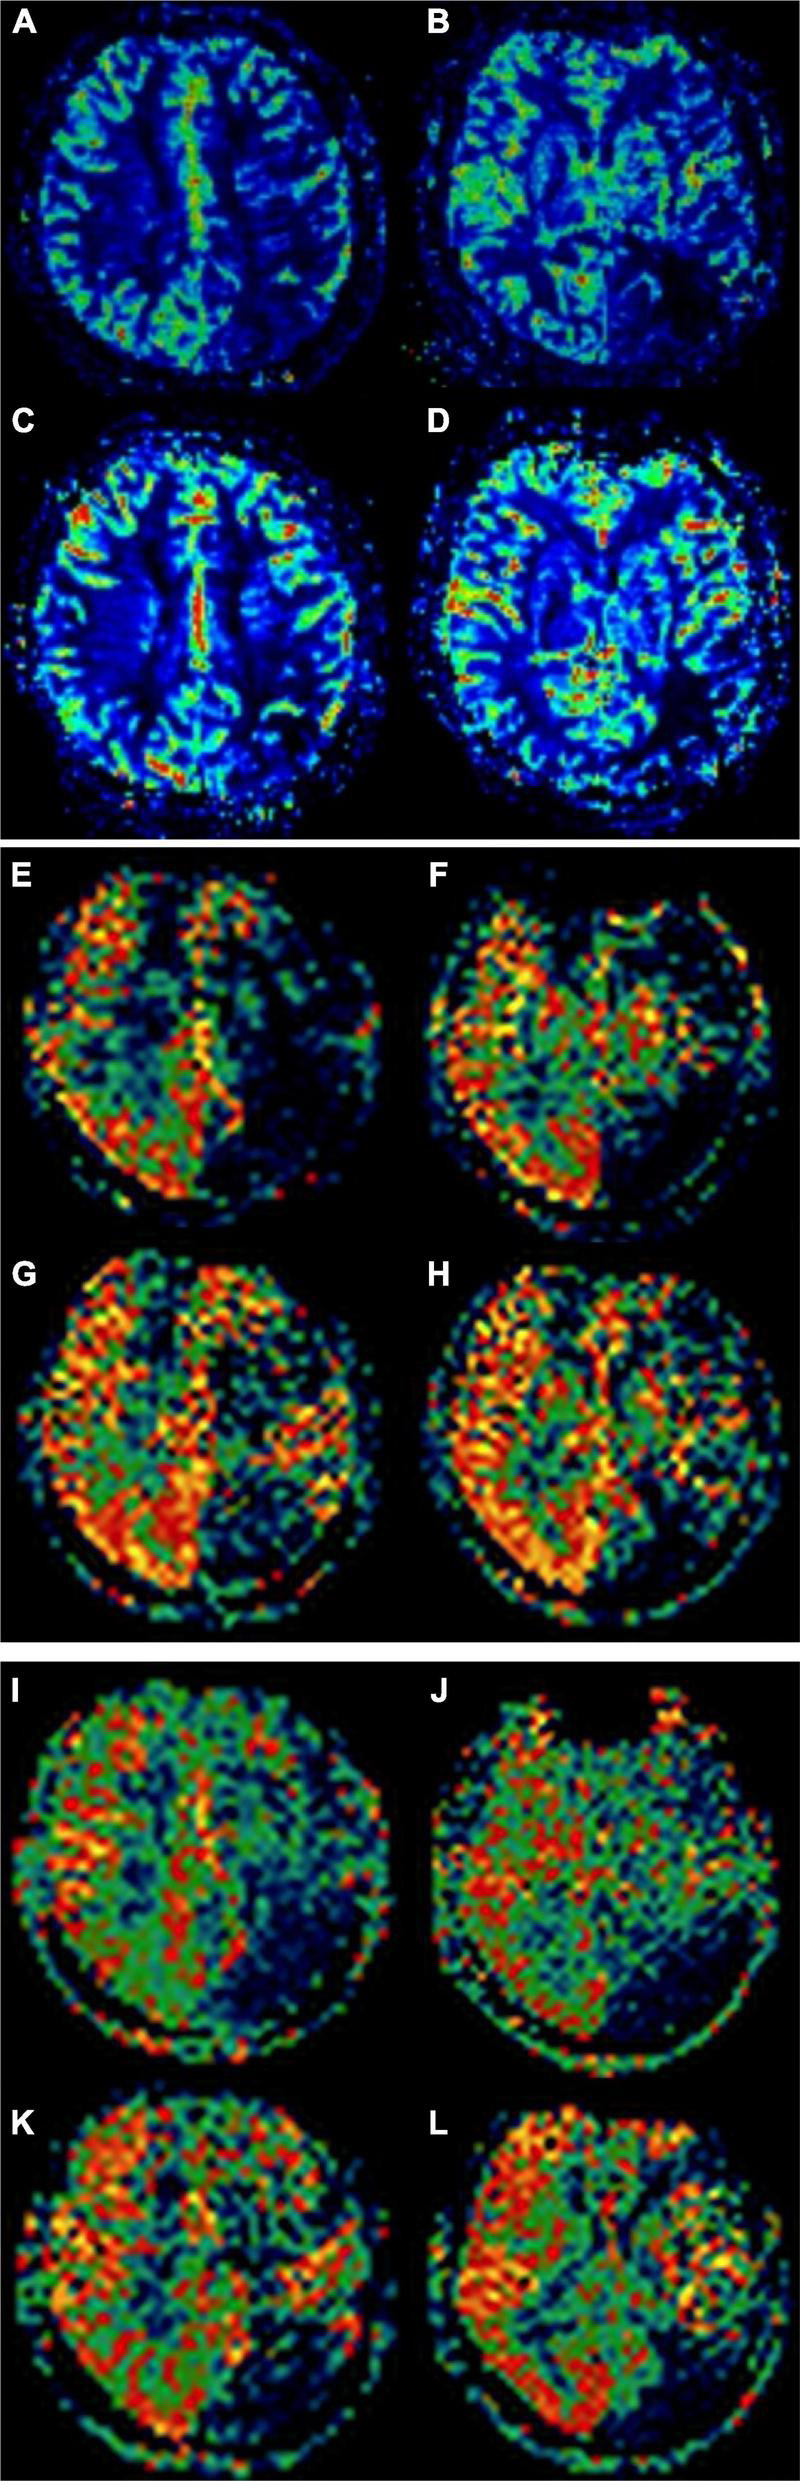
A 51-year-old female patient with moyamoya disease (MMD) underwent encephaloduroarteriosynangiosis (EDAS) on the left side. Alberta Stroke Program Early CT Score (ASPECTS) area ischemia scores of dynamic susceptibility contrast-cerebral blood flow (DSC-CBF) before revascularization were 5 points (A,B). ASPECTS area ischemia scores after revascularization were 8 points (C,D). ASPECTS area ischemia scores of arterial spin labeling (ASL)-CBF (1,500 ms) before revascularization were 5 points (E,F). ASPECTS area ischemia scores after revascularization were 9 points (G,H). ASPECTS area ischemia scores of ASL-CBF (2,500 ms) before revascularization was 4 points (I,J). ASPECTS area ischemia scores after revascularization were 8 points (K,L).
